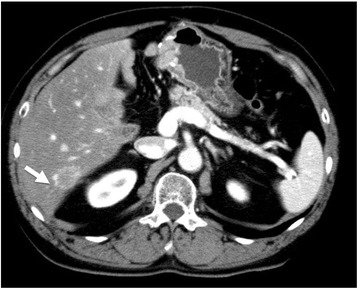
Abdominal computed tomography scan shows liver metastasis in segment 6 (arrow)
